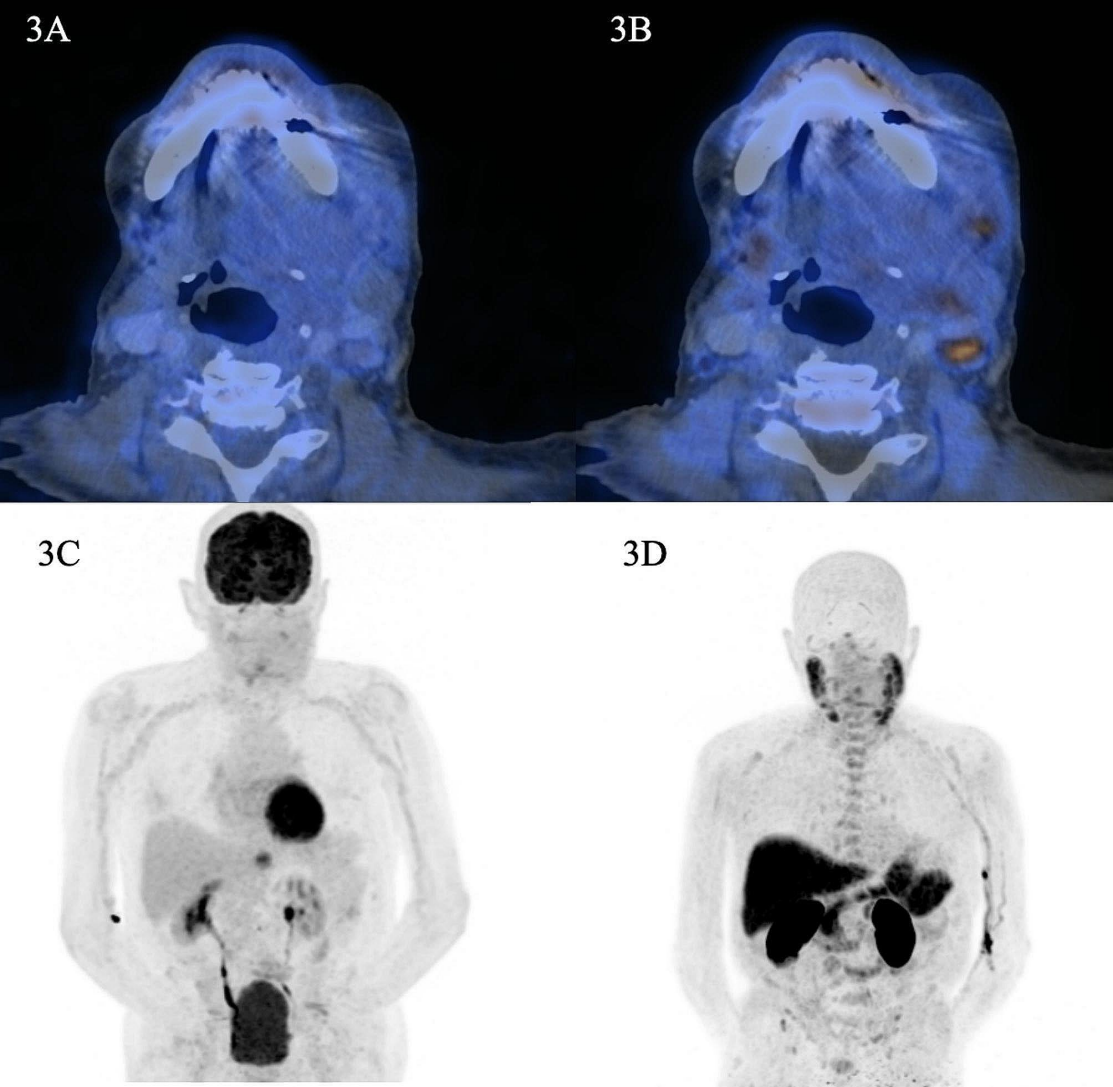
(A) axial hybrid FDG-PET/CT images of patient 3 with high grade adenocarcinoma of the left parotid showing low uptake of the tumor (SUVmax 2.4). (B) axial hybrid choline-PET/CT images of patient 3 with high grade adenocarcinoma of the left parotid, showing moderate uptake of tumor (SUVmax 7.2). (C) maximum intensity projection (MIP) of patient 3 on FDG-PET showing almost no uptake in the parotid gland (and typical uptake in the brain, heart, and lower urinary tract). (D) maximum intensity projection (MIP) of patient 3 on choline-PET showing uptake in salivary glands (and in the tumor) and choline uptake in the liver, pancreas, kidney, and spleen
